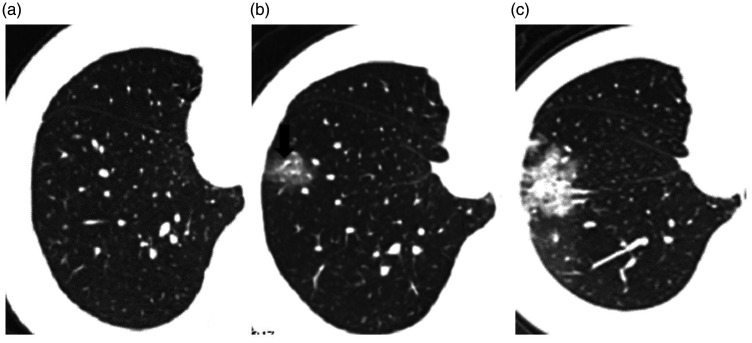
(a) CT scan two days after onset of symptoms; CT is negative. (b) CT scan five days after onset of symptoms, showing GGO with vascular congestion (arrow). (c) CT scan eight days after onset of symptoms, showing GGO with vascular congestion and part consolidation. CT, computed tomography; GGO, ground-glass opacity.
